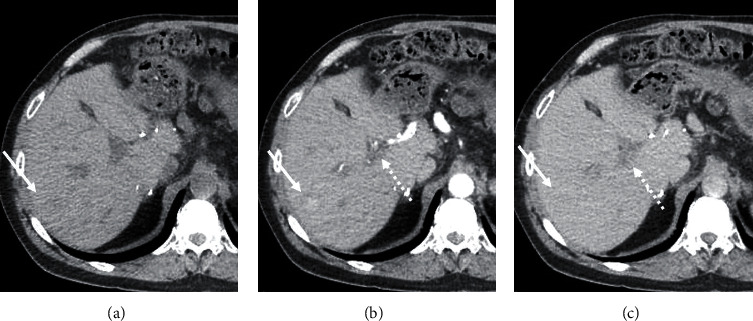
Retrospective review of a contrast-enhanced CT of the liver performed 8 months prior to contrast-enhanced MRI demonstrates portal vein thrombosis (dotted arrow) and a 2.3-cm lesion in segment 7 (arrow) that is faintly hypodense on precontrast (a), enhances centrally with a hypoenhancing rim on arterial phase (b), and subtly hypodense with a subtle rim of hyperenhancement on 3 min delay phase (c). In a patient with chronic liver disease, this appearance would be suspicious for HCC.
